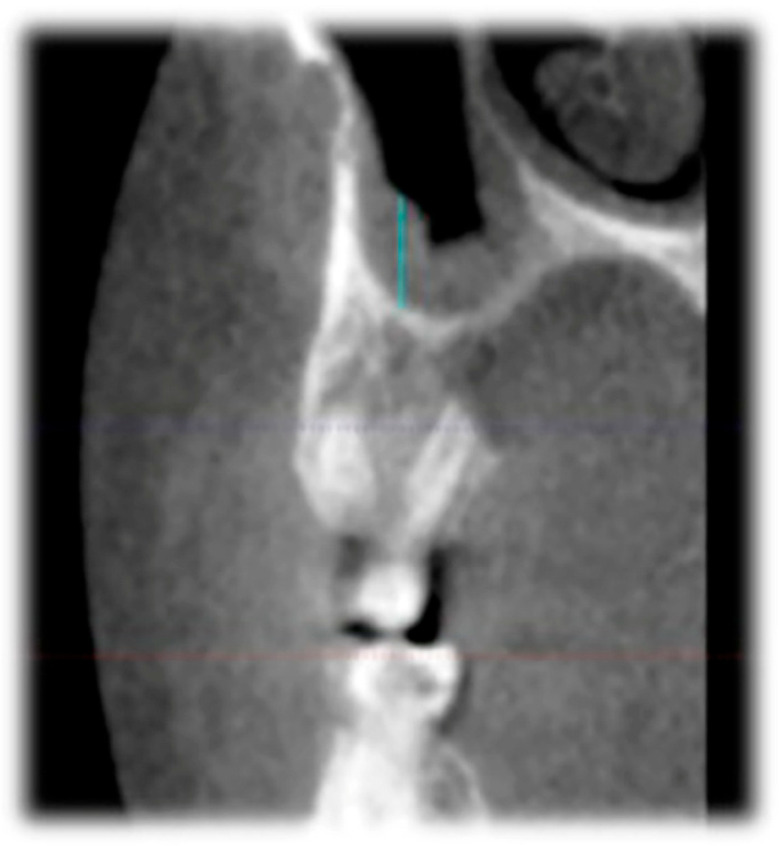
Measurement of MT on a sagittal CBCT image. The blue vertical line represents MT, measured from the bony floor of the maxillary sinus to the outermost border of the Schneiderian membrane. This value reflects the maximum MT in the evaluated region. CBCT enables precise identification of anatomical boundaries, facilitating reliable assessment of sinus membrane thickening potentially associated with periodontal or periapical pathology. In Figure 2, mucosal thickening was differentiated from mucus retention or other sinus contents by its continuous, uniform, and well-defined radiopaque appearance along the bony sinus wall. In contrast, mucus or fluid accumulation typically presents with different radiodensity patterns, lacks a consistent attachment to the sinus wall, and may exhibit air-fluid levels. Only cases with clearly demarcated mucosal thickening were included in the measurements.
